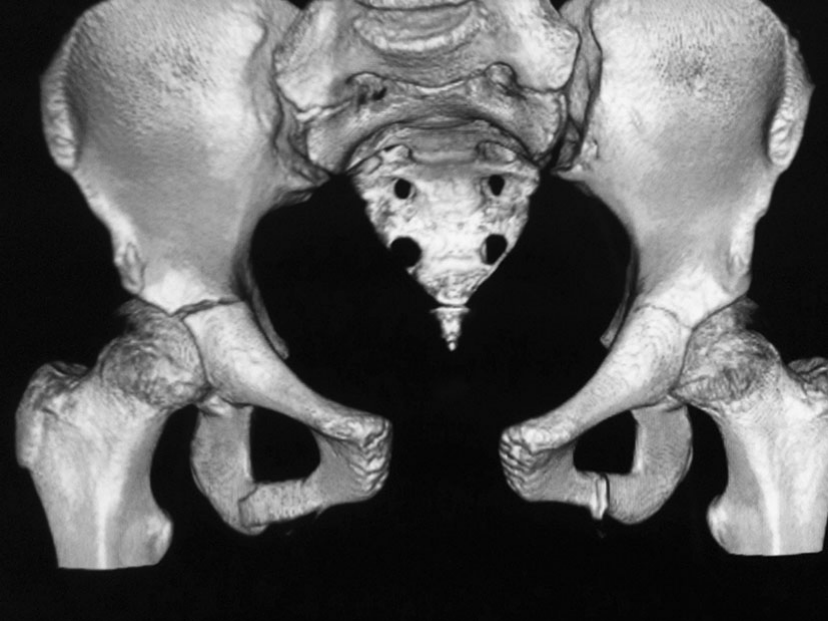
3D CT of pelvis: integrity of both sacroiliac joints, symphysis diastasis (Tile B1) and damage to triradiate cartilage at right (type I of Salter–Harris)
